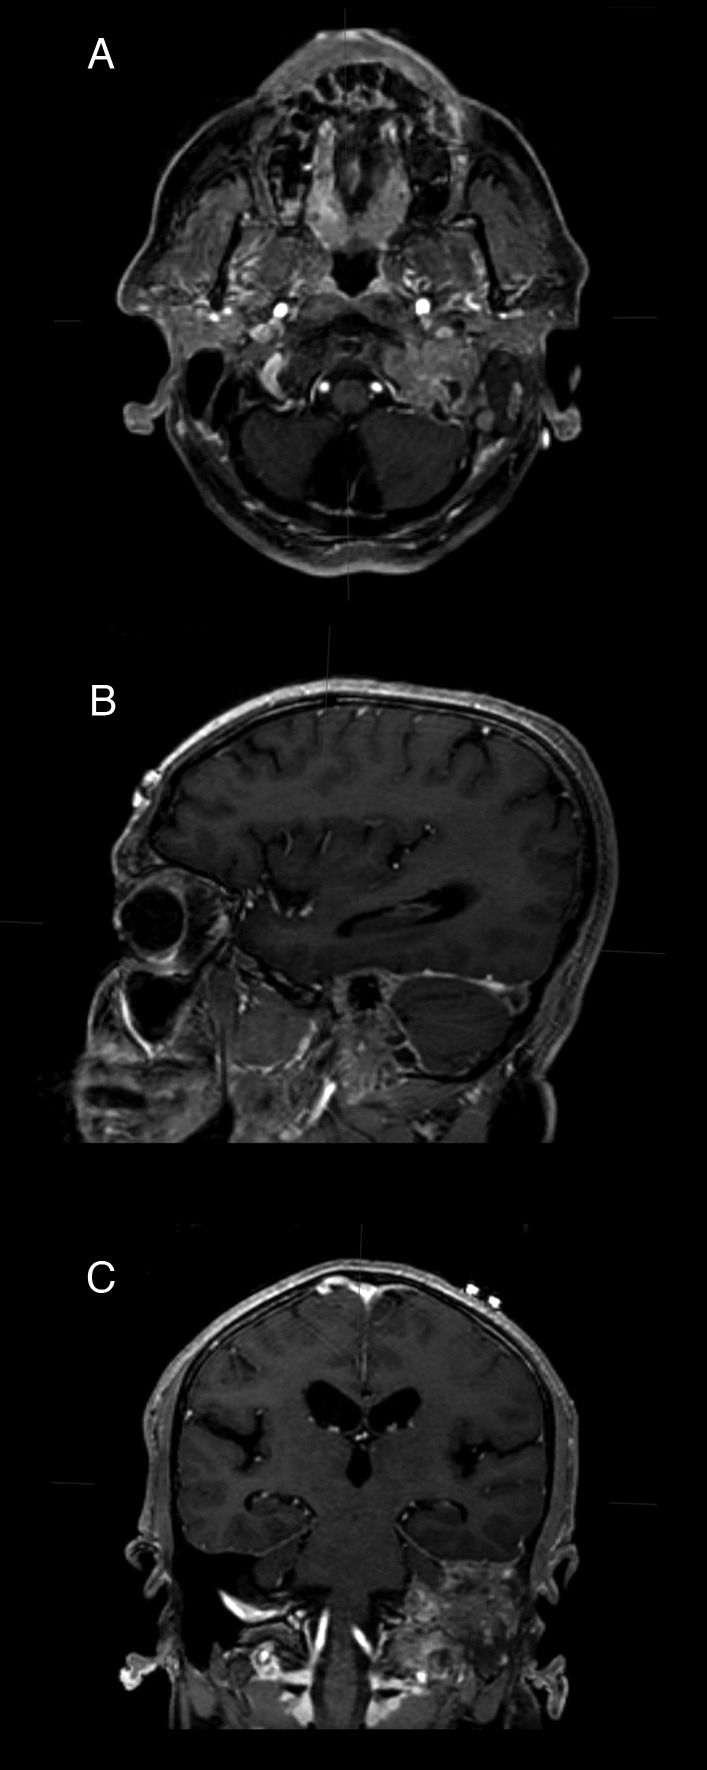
a Axial, b sagittal, and c coronal T1-weighted magnetic resonance images of the tumor revealing a lobulated, rather well-demarcated isointense to hyperintense mass centered in the right jugular fossa infiltrating into the posterior fossa and temporal bone
